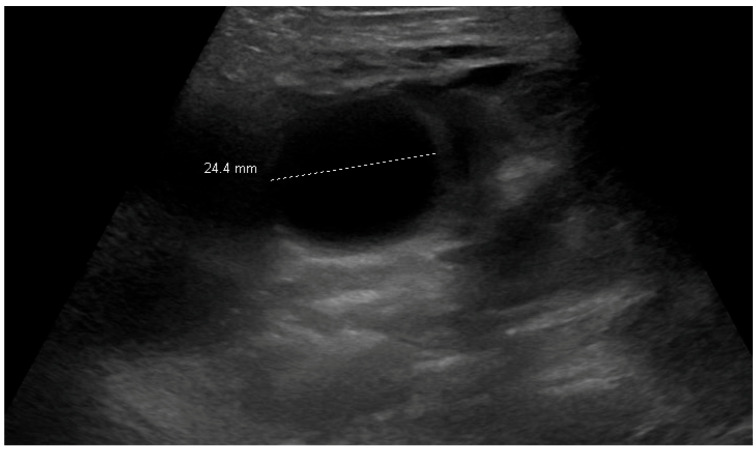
Ultrasound image obtained on postoperative day 4, depicting a rounded foreign body within the intestine.
